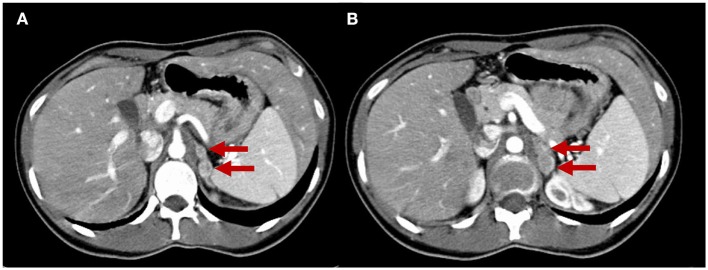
Adrenal CT scan. The two slices (A,B) show four nodules in the left adrenal gland measuring from 10 to 18 mm that are unattenuated after contrast administration (red arrows), compatible with left multifocal adrenal pheochromocytomas.
